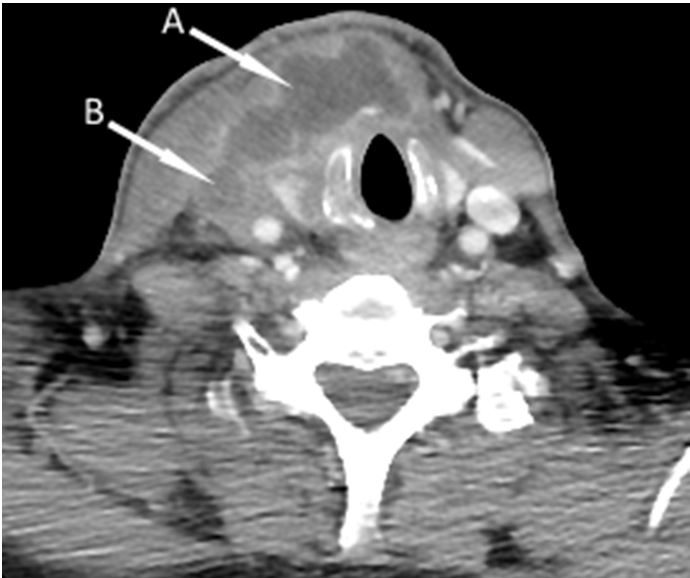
Contrast-enhanced computed tomography of the neck showing a soft tissue fluid collection (A) anterior and lateral to the trachea, and thrombus in the right internal jugular vein (B)
